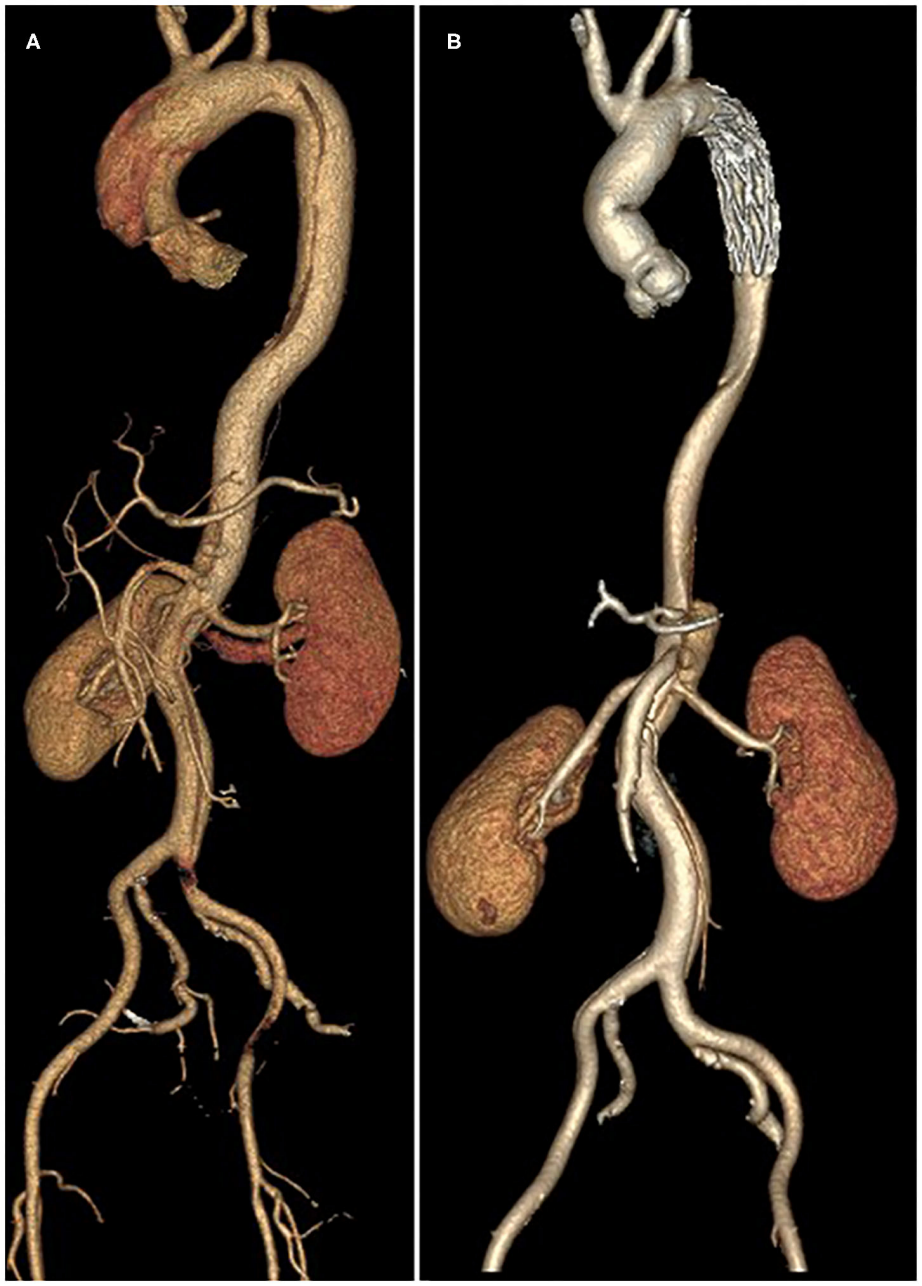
Preoperative (A) and postoperative (B) computed tomography angiography reconstruction.
